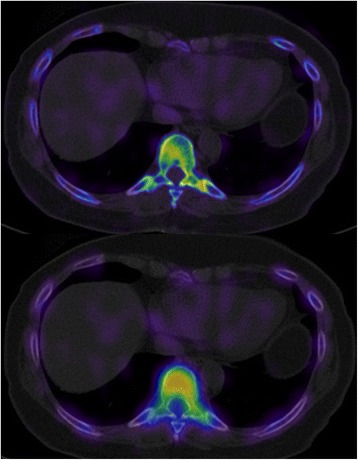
Costovertebral joint uptake in xSPECT/CT v SPECT/CT. Detailed legend: The upper image shows a fused xSPECT-CT transaxial image while the lower image shows a fused SPECT-CT image (same slice in the same patient). Note the clear abnormality identified in the costovertebral joint on xSPECT, which is not identified on SPECT
